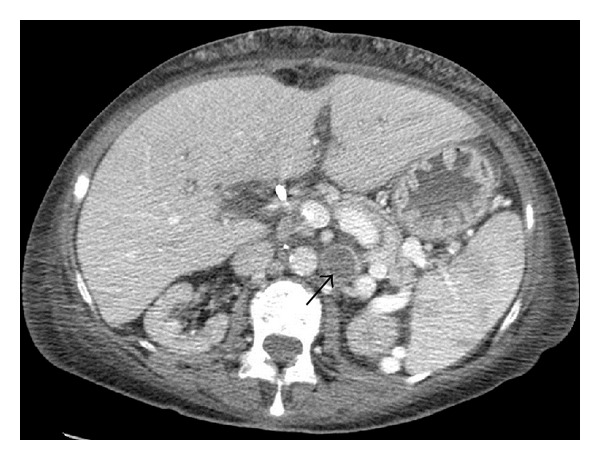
Postoperative CT imaging (day 47) showing the thrombosis of the left renal vein at the point of ligation (arrow).
